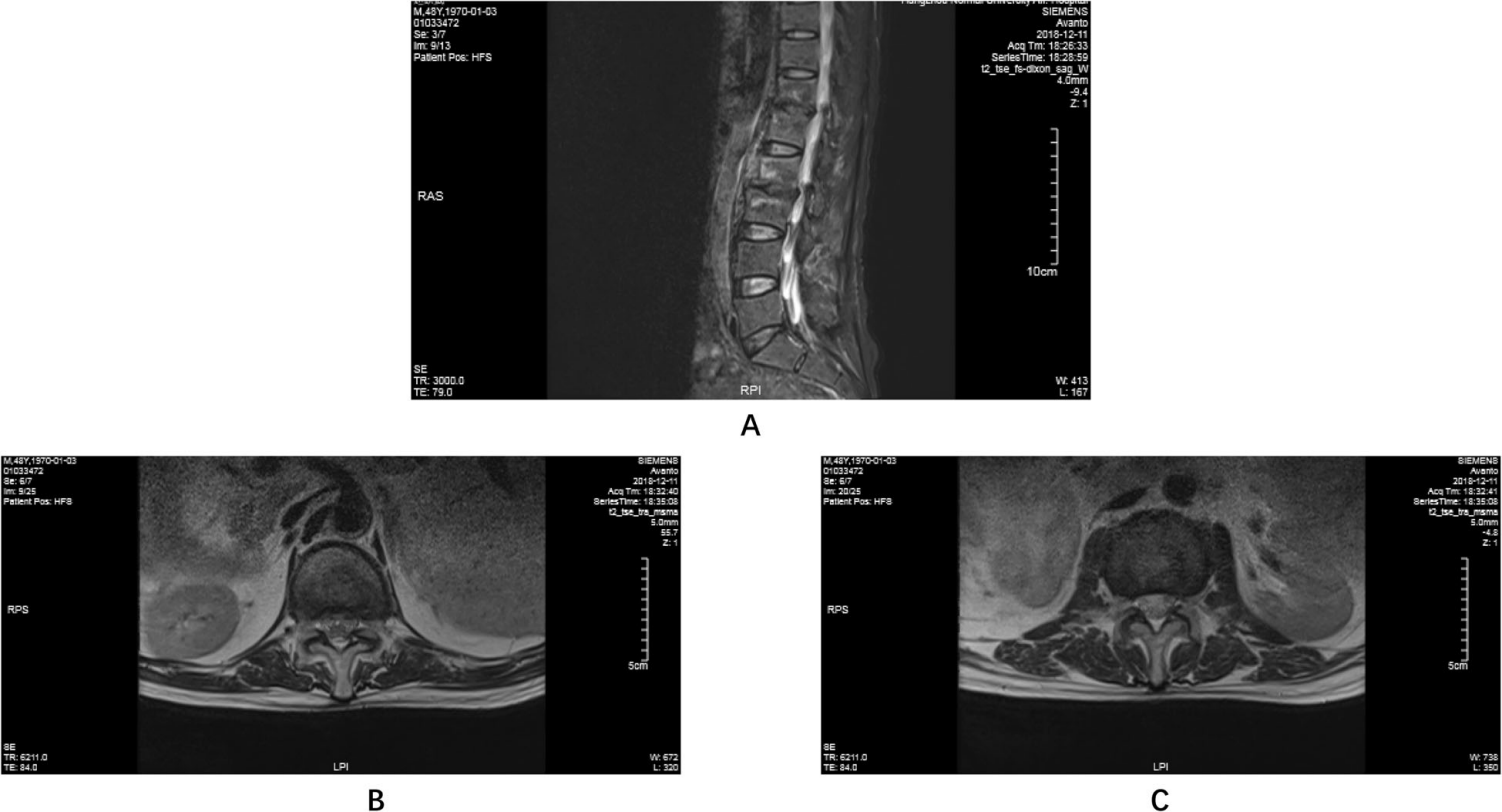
Lumbar MRI (2018-12-11) a sagittal plane. b transverse section of T12/L1. c transverse section of L2/L3
